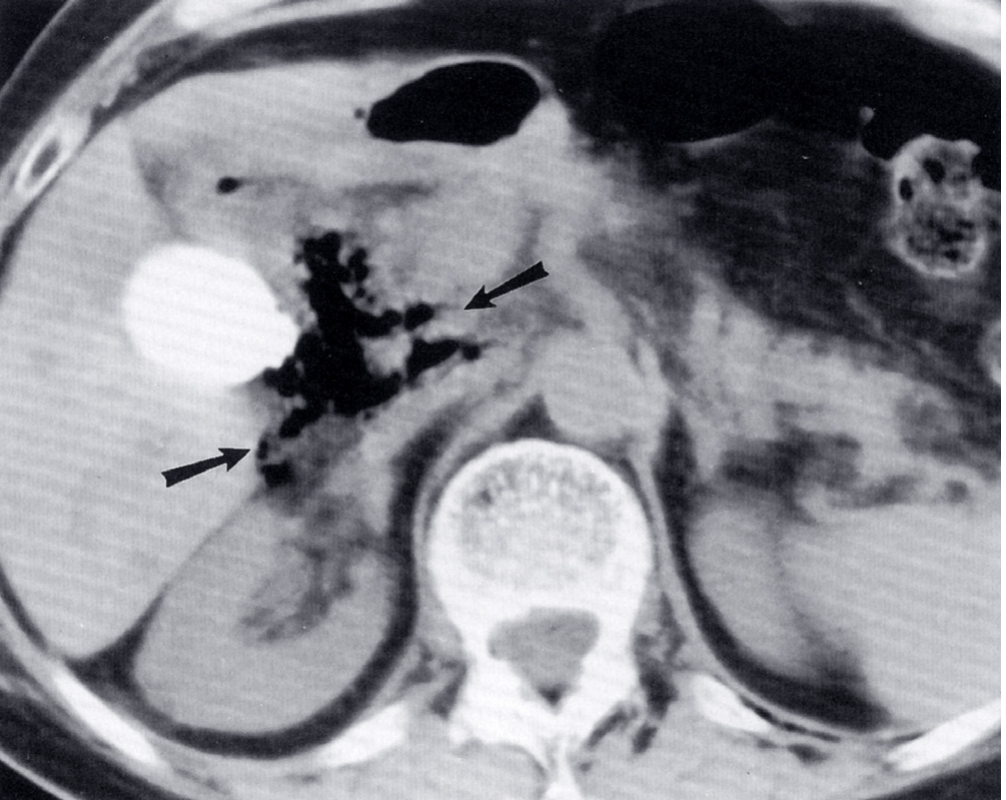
Radiograph showing air (arrow) in the retroperitoneal space.
